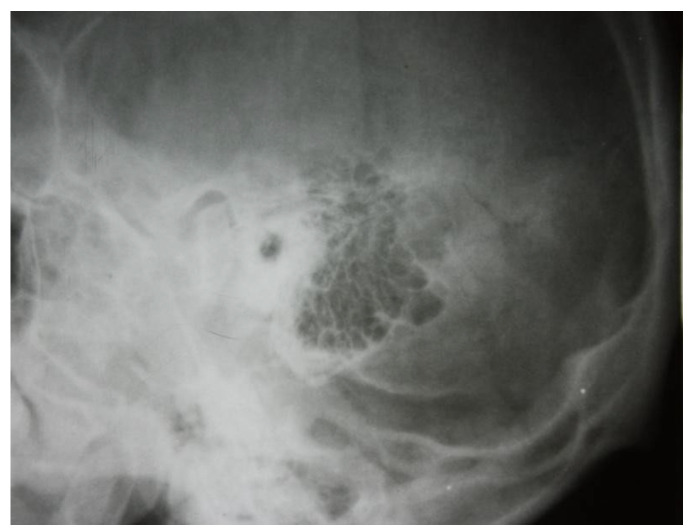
A radiograph of the left petromastoid region in the temporo-tympanic (Schüller) incidence showed reduced transparency of most mastoid cells.
